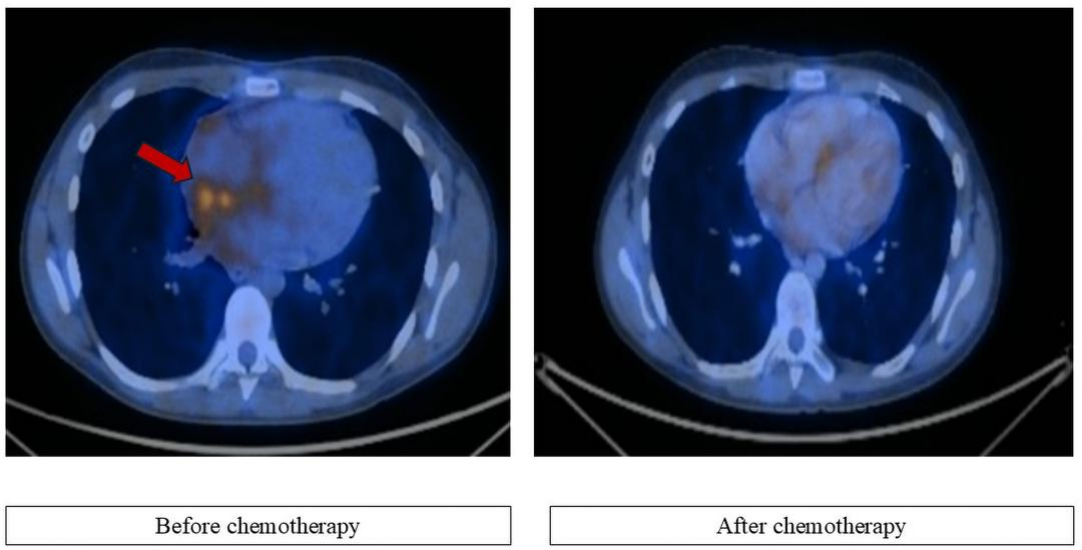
Cardiac nuclear imaging investigation; PET-CT scan before and after initiation of chemotherapy; red arrow indicates extensive FDG uptake predominantly at RA level, with a clear decrease after chemotherapy.
